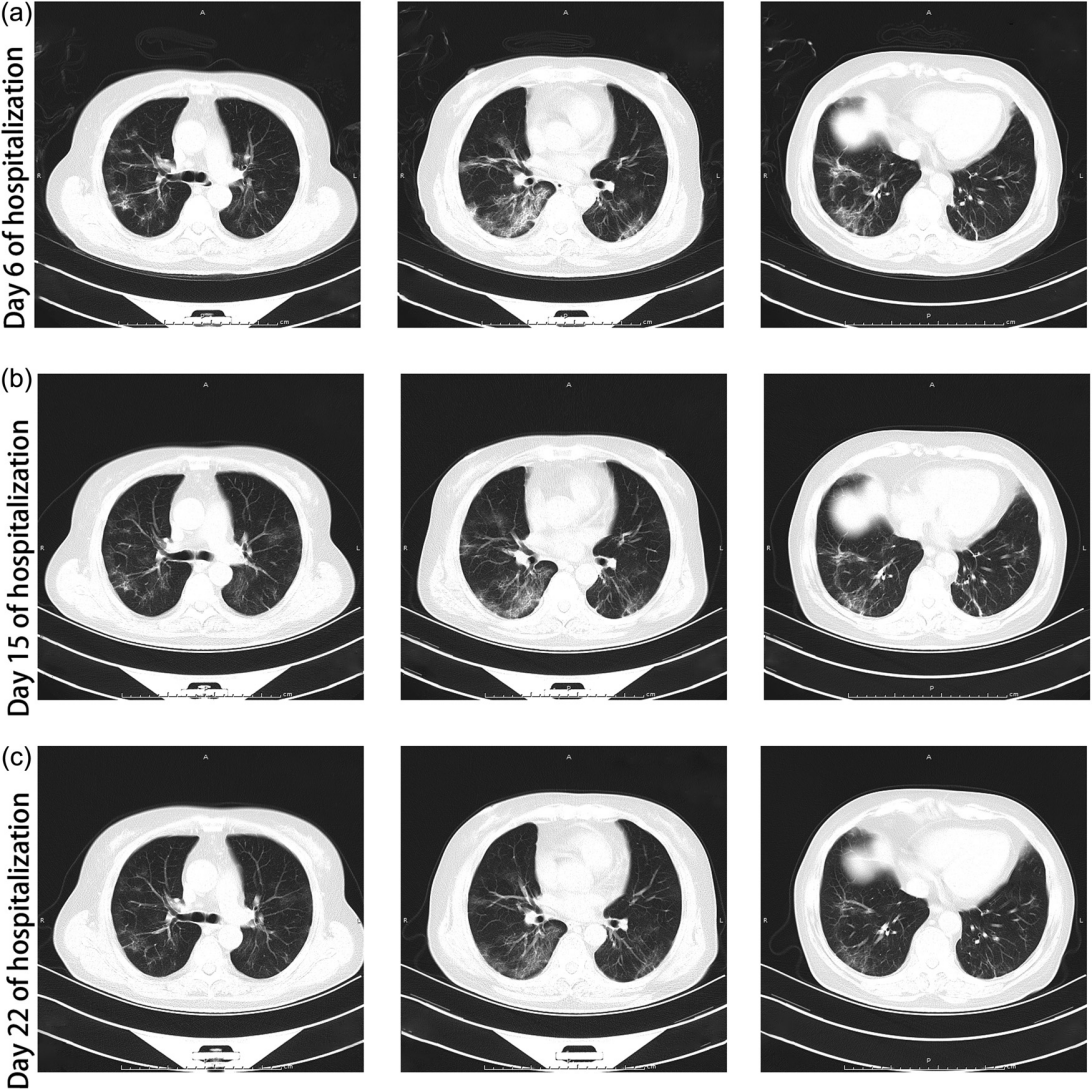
Thoracic CT images. (a) Thoracic CT of day 6 of hospitalization showed bilateral ground-glass, patchy and cord-like shadows. (b) Thoracic CT of day 15 of hospitalization showed that pulmonary infiltration was partly absorbed. (c) Thoracic CT of day 22 of hospitalization showed that pulmonary infiltration was absorbed obviously.
